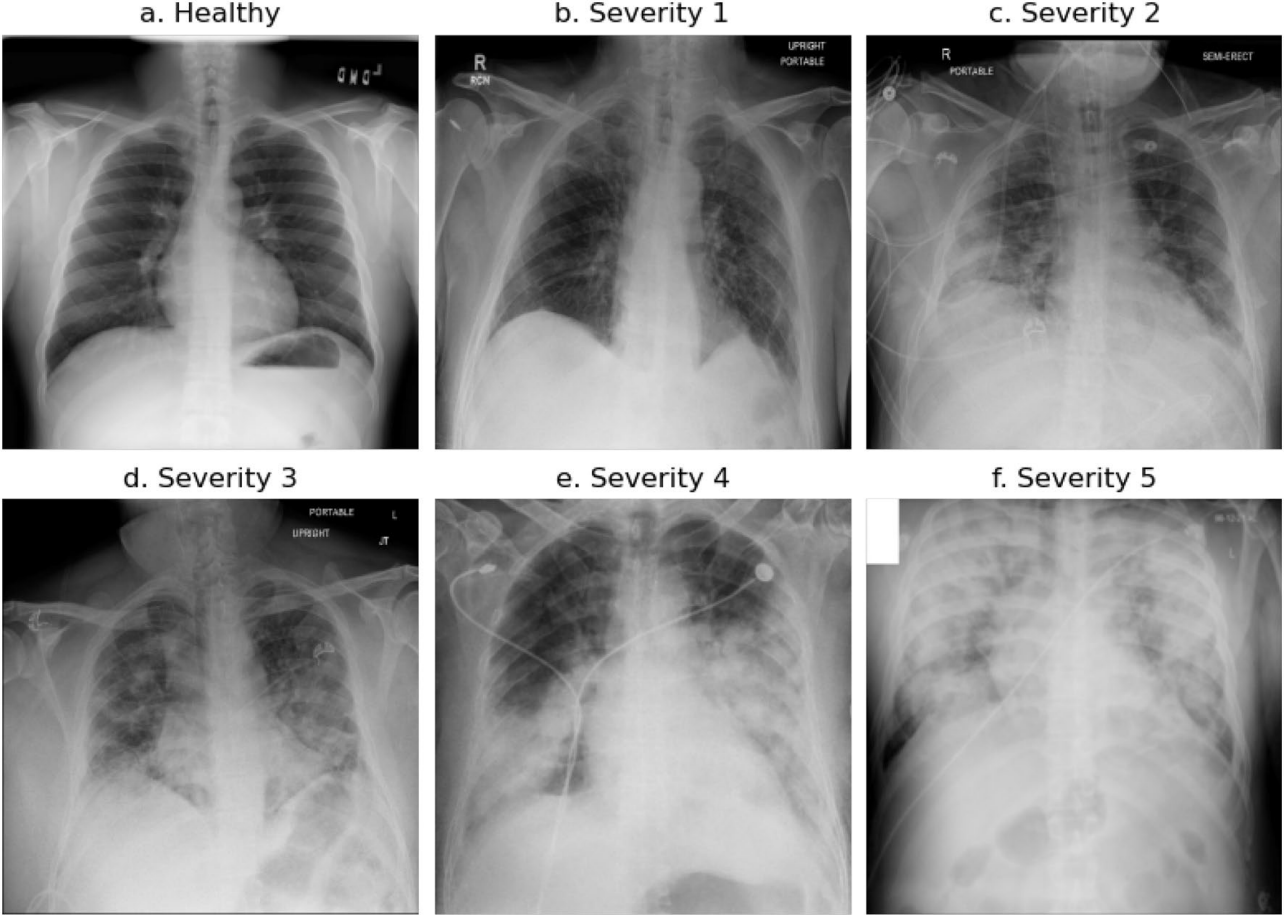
Example chest X-ray images of healthy patient (a) and severity scoring 1–5 (b–f).
